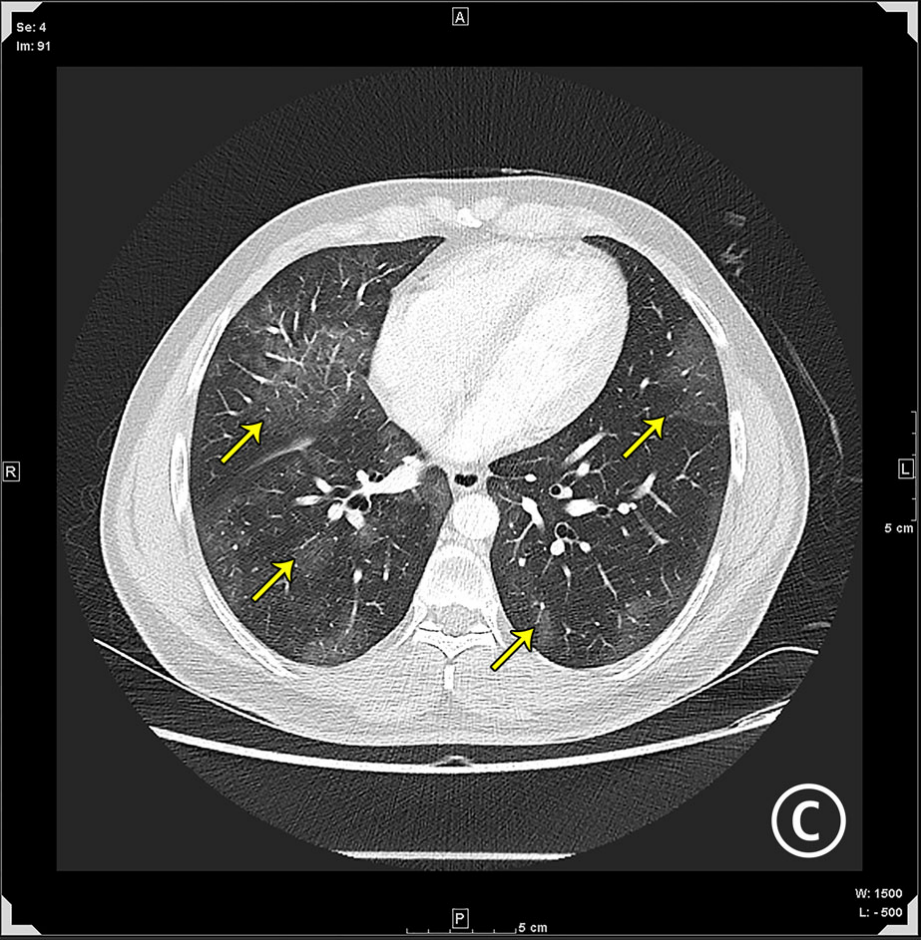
Computed tomography thorax in April 2021 shows bilateral new‐onset ground‐glass opacities
